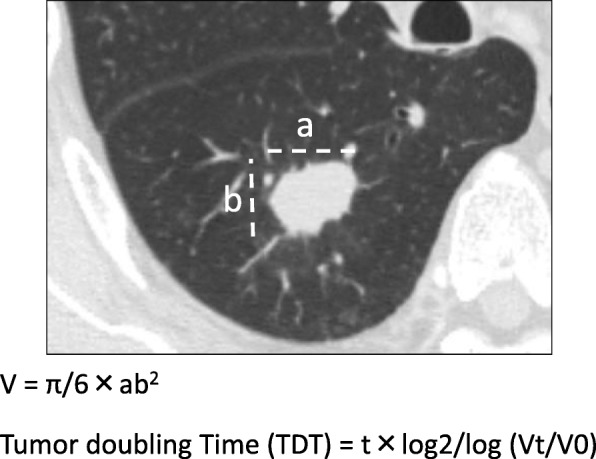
CT findings and the Schwartz equation used to determine tumor volumes. a, maximum tumor diameter; b, largest perpendicular tumor diameter; t, time between the two CT scans; Vt, tumor volume from preoperative CT scan; V0, tumor volume from initial CT scan
